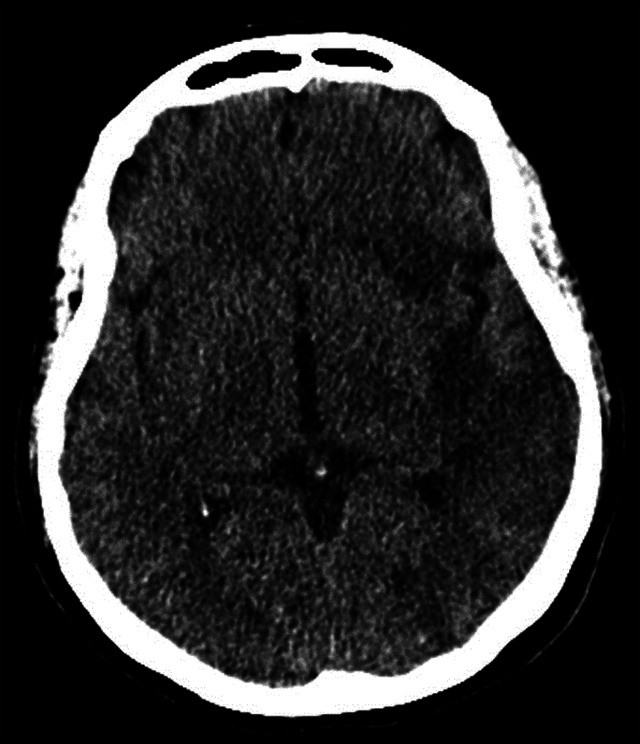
Follow-up head CT depicts the ischemic area in the left hemisphere more clearly as a hypoattenuation involving the left insula and frontal lobe.
